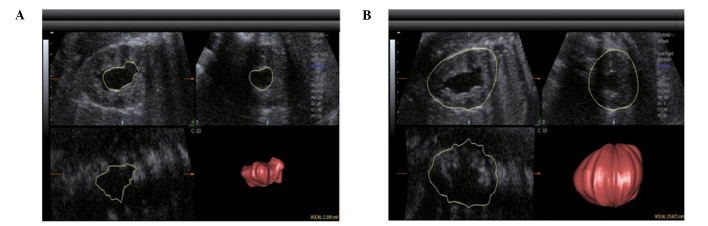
Ultrasound images showing (A) kidney volume V1 and (B) dilated renal pelvis volume V2. The renal parenchymal volume/kidney volume, (V1−V2)/V1 = (25.671–2.109)/25.671 = 0.92.
